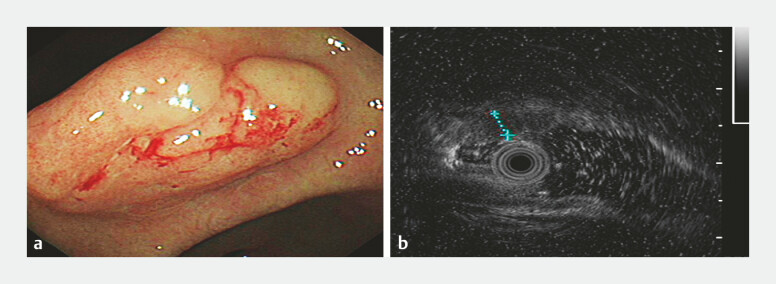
Endoscopic and ultrasound findings of a gastric lesion at the gastric angle.
